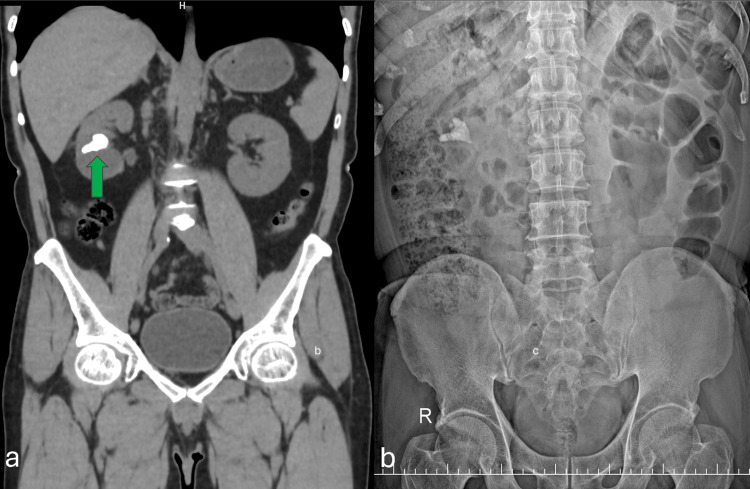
Coronal CT and plain abdominal radiograph demonstrating urinary tract calculi. (a) Coronal non-contrast CT of the abdomen reveals a large radiodense calculus within the left renal pelvis (green arrow). (b) Corresponding plain abdominal radiograph (kidney, ureter, bladder) shows radiopaque shadows consistent with urinary calculi in the left kidney.
